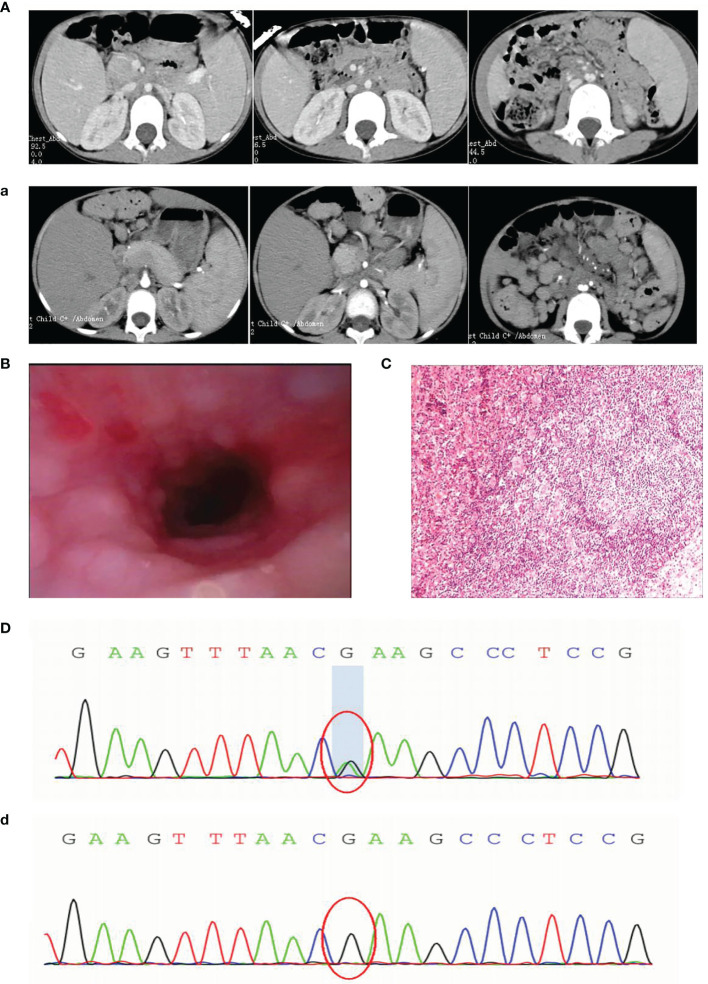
(A) First CT: enlarged lymph nodes (a) 1 year later CT: extensive lymph node enlargement (B) Fibroscopy: numerous nodular protrusions (C) Lymph node biopsy: EBV-associated lymphoproliferative lesions (EBER+) (D) Sanger sequencing images before transplantation (d) Sanger sequencing images after transplantation.
